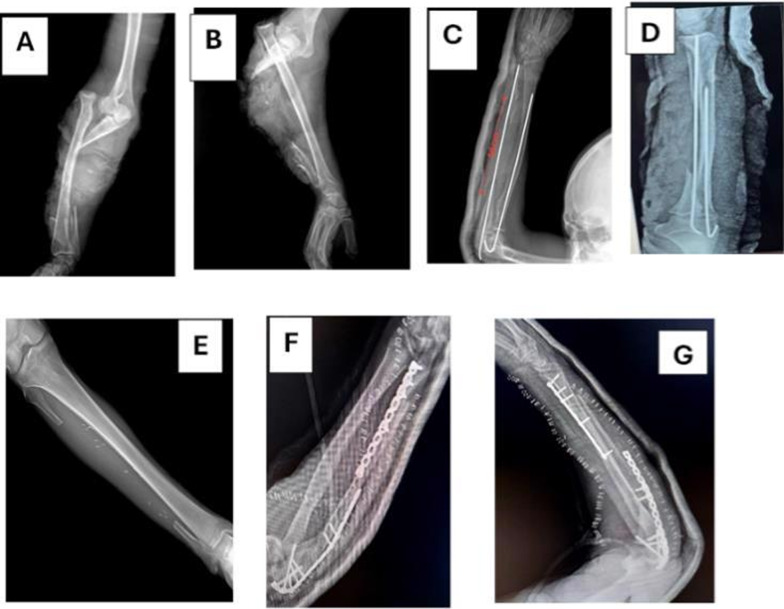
A, B Pre-x-ray, C, D 1st stage postpreliminary fixation by k-wire and reduction of radial head, E x-ray leg postharvest free fibula, F, G immediate postoperative x-ray
